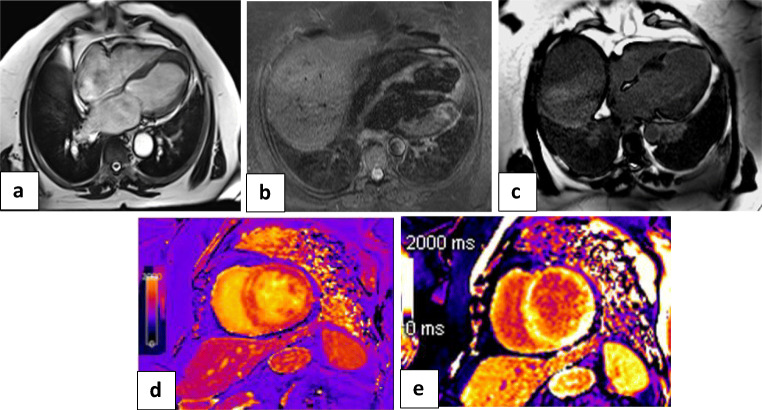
Cardiac MRI: a White blood image 4-chamber view showing ballooning and thinning out of the cardiac apex with apical thrombus. b T2-weighted-STIR image 4-chamber view showing faint interstitial edema of the cardiac apex and apical cardiac segments. c IR post-contrast image 4-chamber view showing sub-endocardial / trans-mural enhancing zones of the late gadolinium enhancement along with the LAD territory. d, e T2 and T1 cardiac MRI mapping sequence in two-chamber view respectively showing mild interstitial myocardial edema (native T2 = 58 ms) and elevated T1 value = 1193 ms. Conventional coronary angiography of LAD confirmed tapering and narrowing of distal LAD. The LCX and RCA were normal. The final diagnosis was acute coronary syndrome due to coronary vasculitis or oxygen demand mismatch
